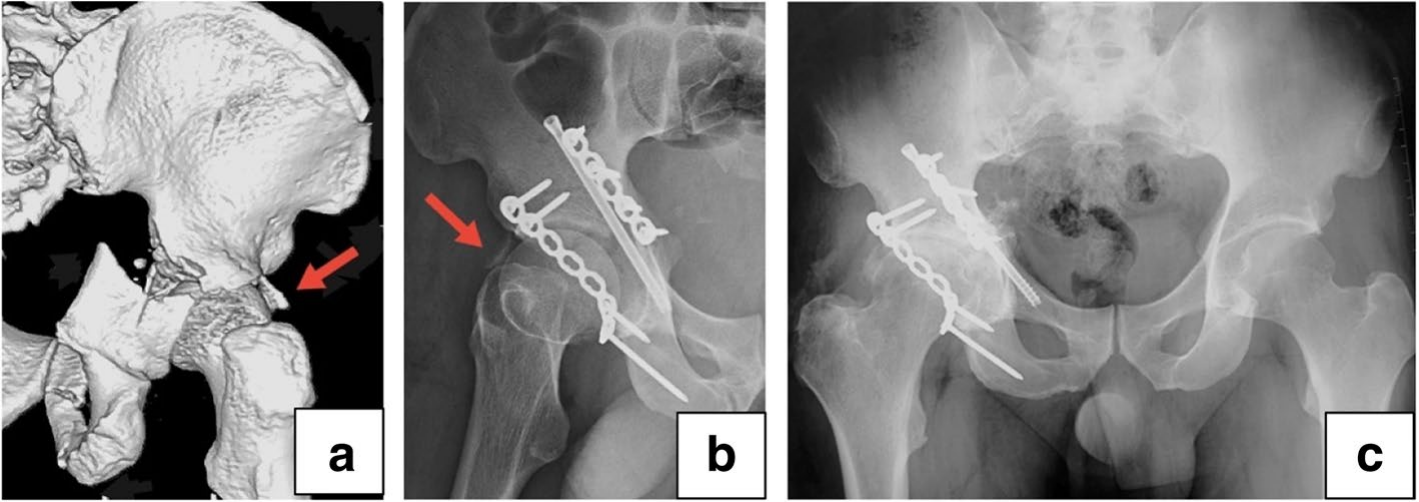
The complication of heterotopic ossification in one case. a An isolated fragment is located at the lateral side of the femoral head (red arrow). b A postoperative radiograph shows that the fragment was not removed (red arrow). c An antero-posterior view shows heterotopic ossification with grade II at the affected hip joint at one-year follow-up
